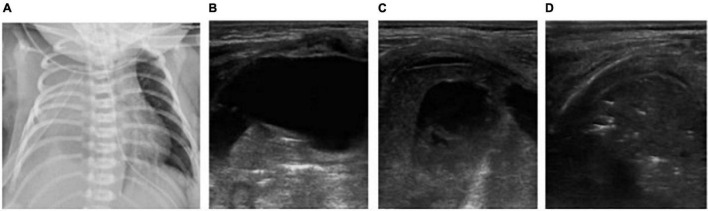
The patient was born at 36^+2^ weeks of gestational age and diagnosed as congenital esophageal atresia. After surgical treatment, man-machine incongruity appeared under mechanical ventilation. X-ray shows white lung on the right lung (A). Atelectasis? or pleural effusion? X-ray failed to distinguish. But LUS clearly showed a large amount of pleural effusion on the right side, the right lung was compressed, showing a liver like echo and the strip bronchial inflation sign, suggesting atelectasis of the right lung (B–D). After thoracic puncture and drainage and adjustment of thoracic drainage tube, the right atelectasis improved.
